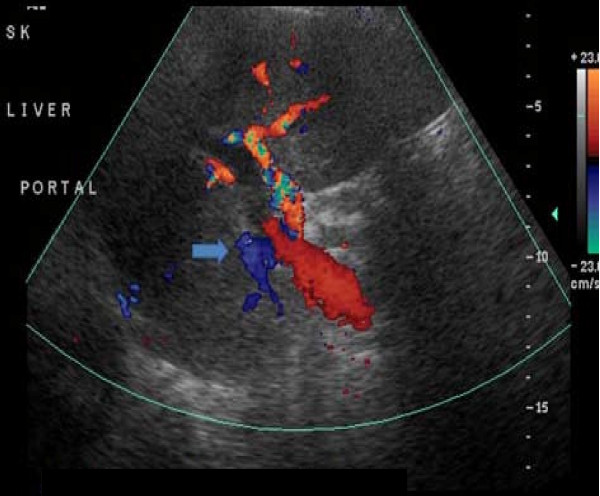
Color Doppler showing no flow in right portal vein.
